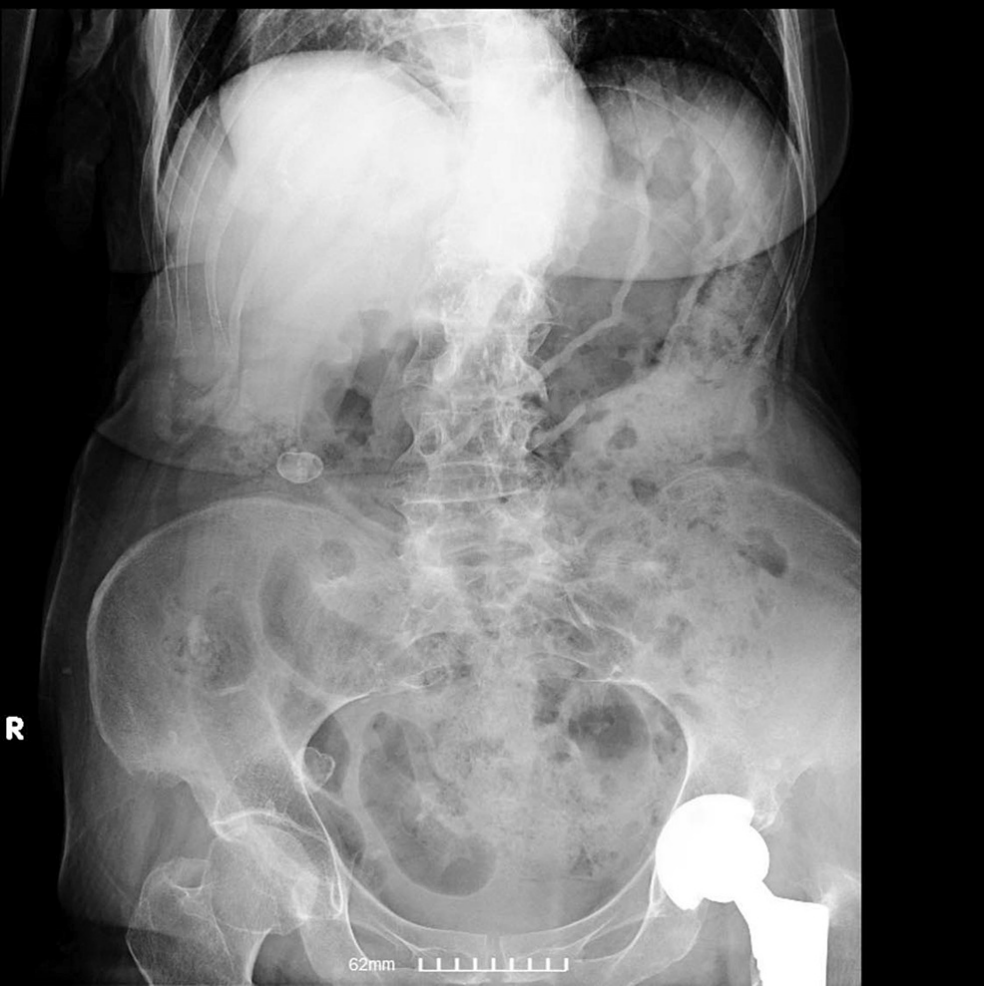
Abdominal X-ray showing dilated loops of bowel.
